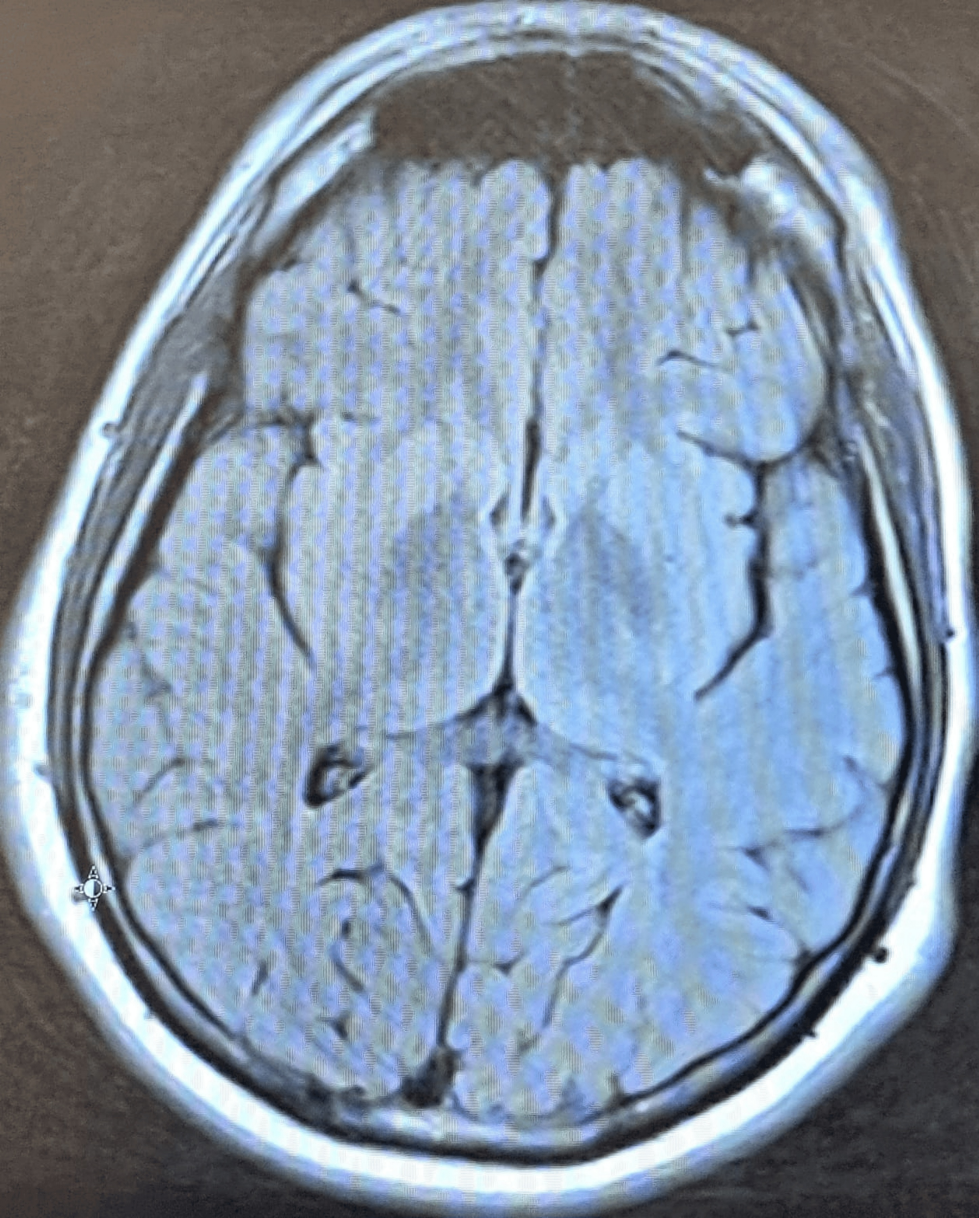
The MRI of Case 2's brain (axial FLAIR image) shows no gross pathology. FLAIR: fluid-attenuated inversion recovery
